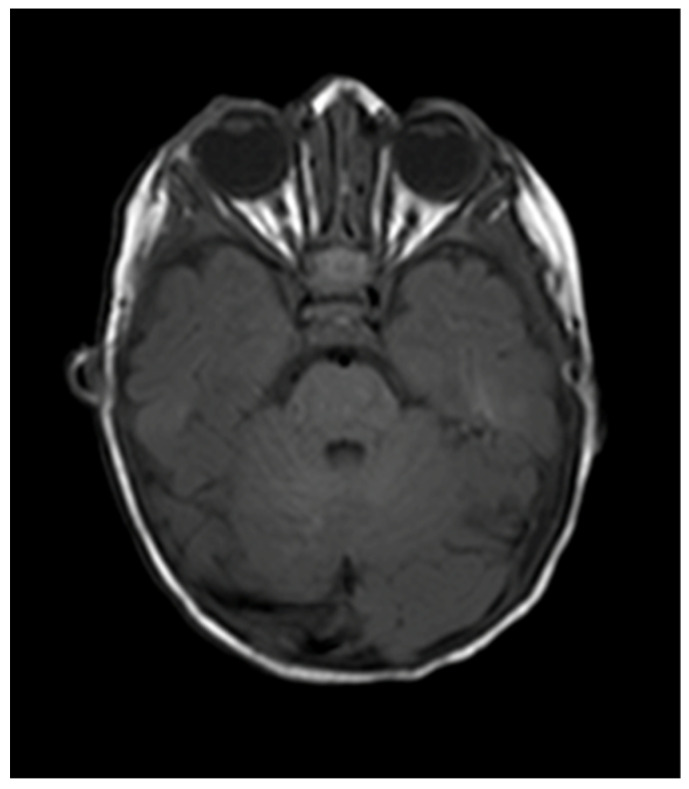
MRI 4 months following commencement of treatment with beta blockers. Total resolution of the orbital hemangioma and of the associated intracranial extension.
